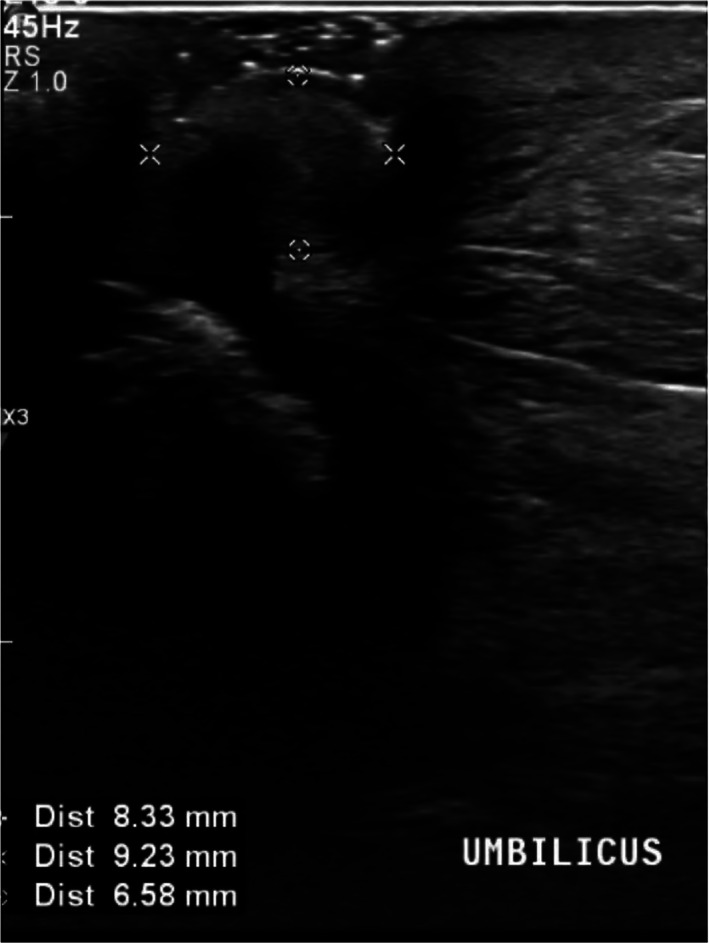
Ultrasound image of the umbilicus showing an isoechoic ovoid focus measuring 8 × 9 × 7 mm, with no associated hernia.
